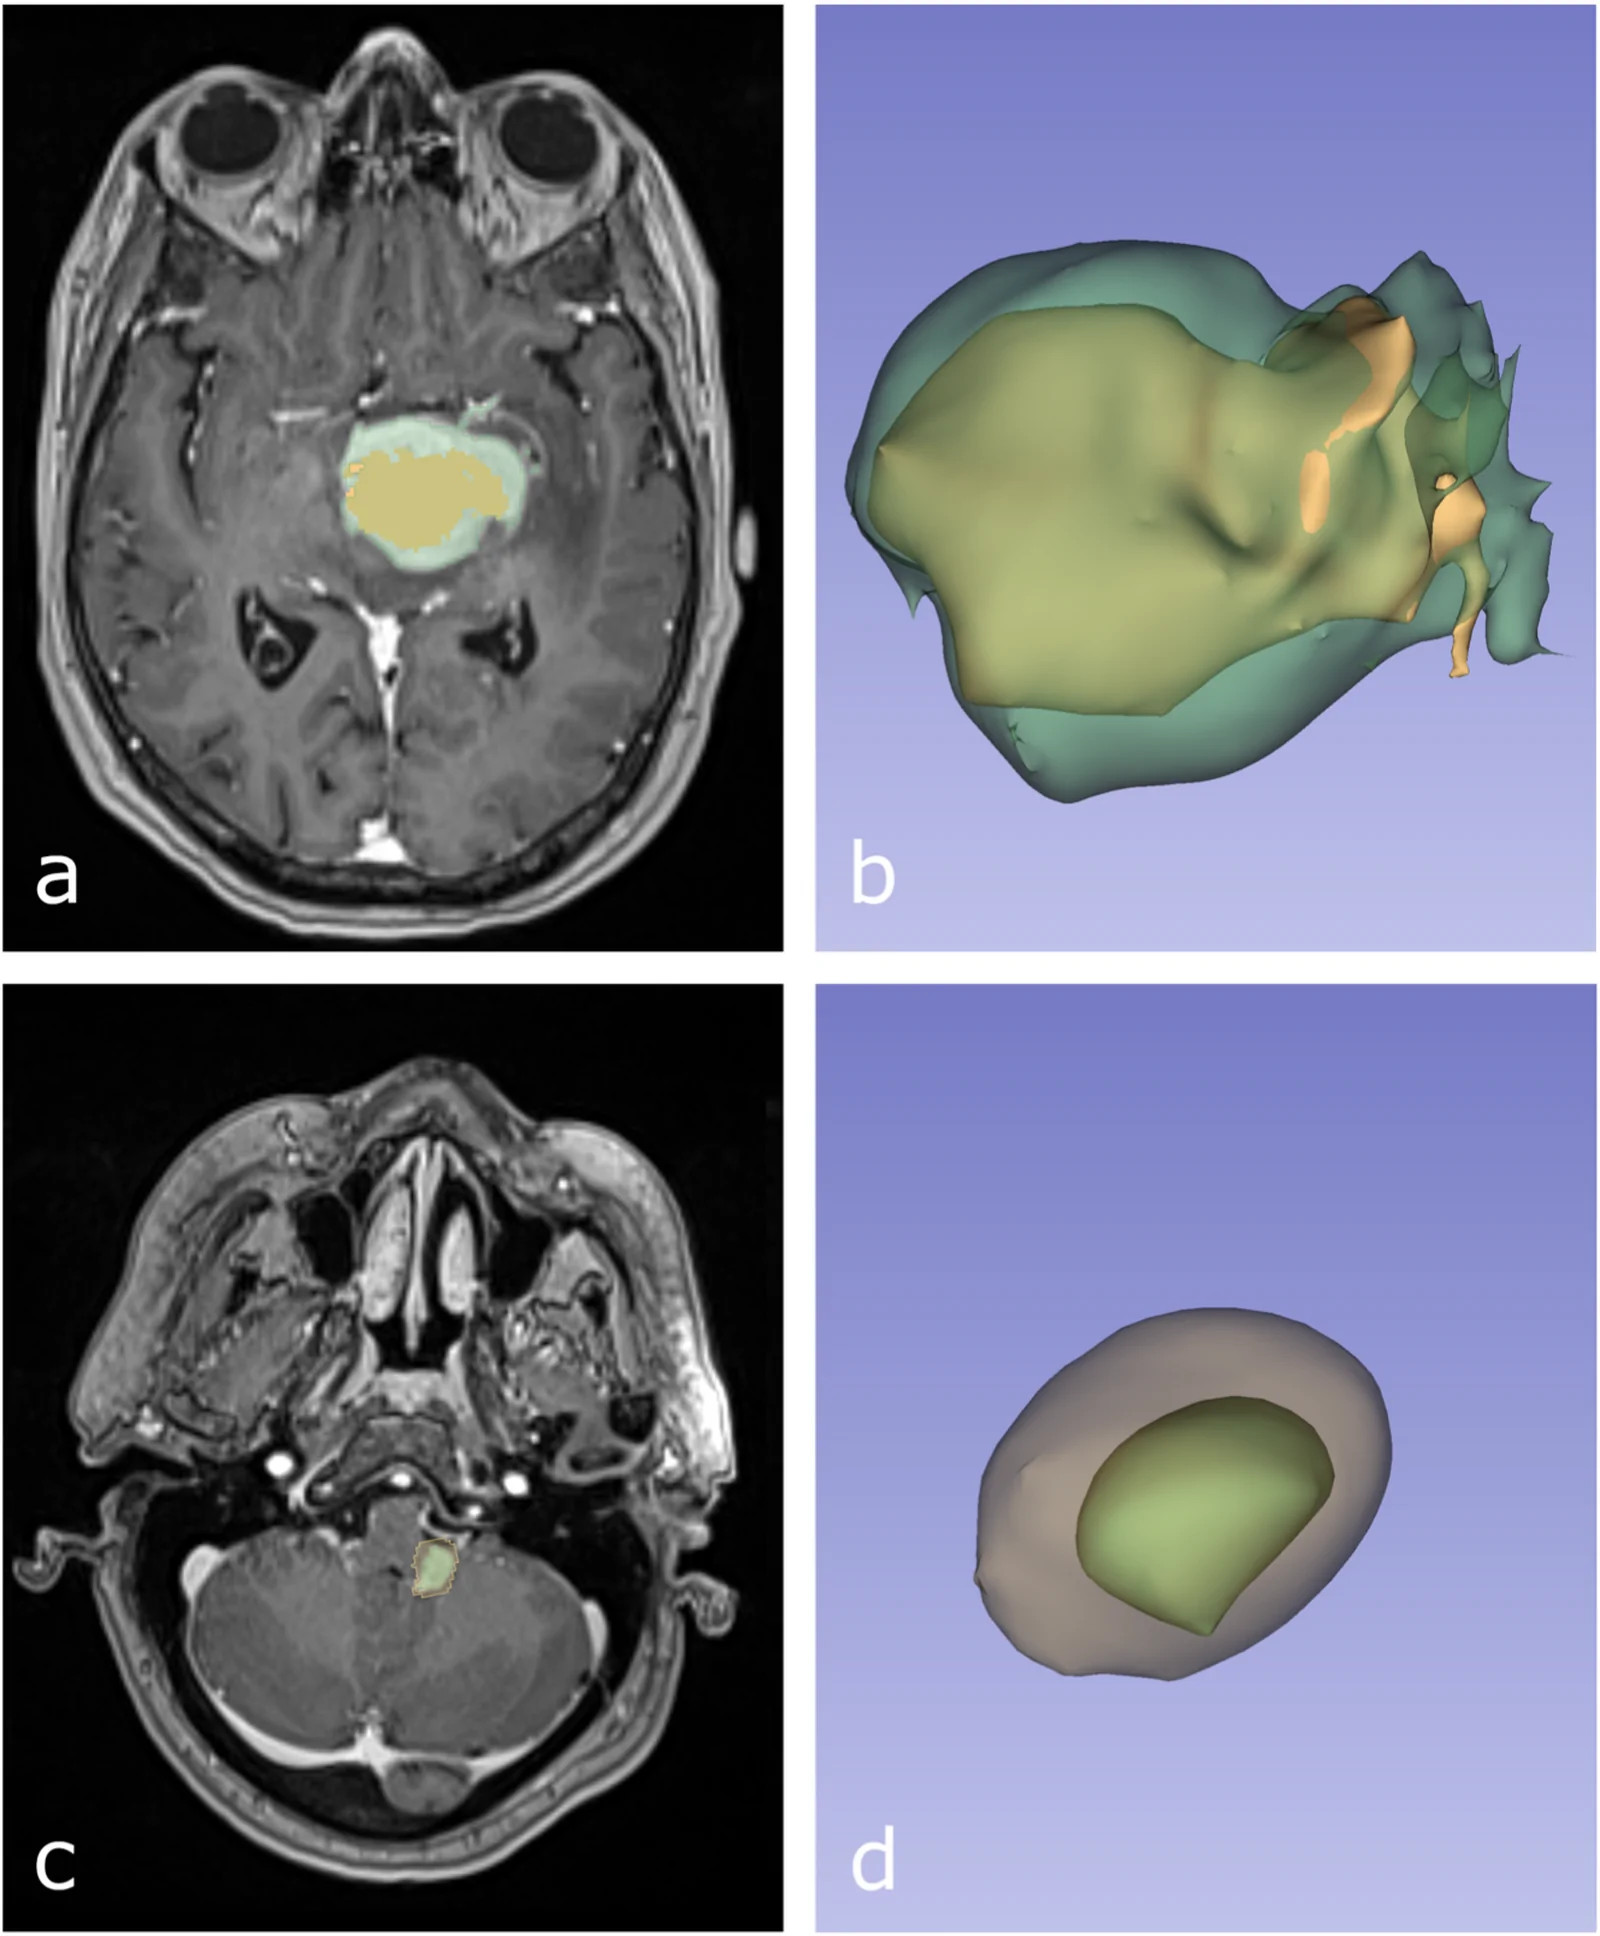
Volumetric analysis of tumor volume; the upper images show a case in which the disease progressed despite CST, while the lower images show regression following CST. a and c: post-CST axial slice of T1 MRI with contrast enhancement showing the tumor after CST. Yellow marks the corresponding preoperative lesion before corticosteroids and green the tumor after CST. b and d: volumetric rendering of preoperative and post-CST tumor
